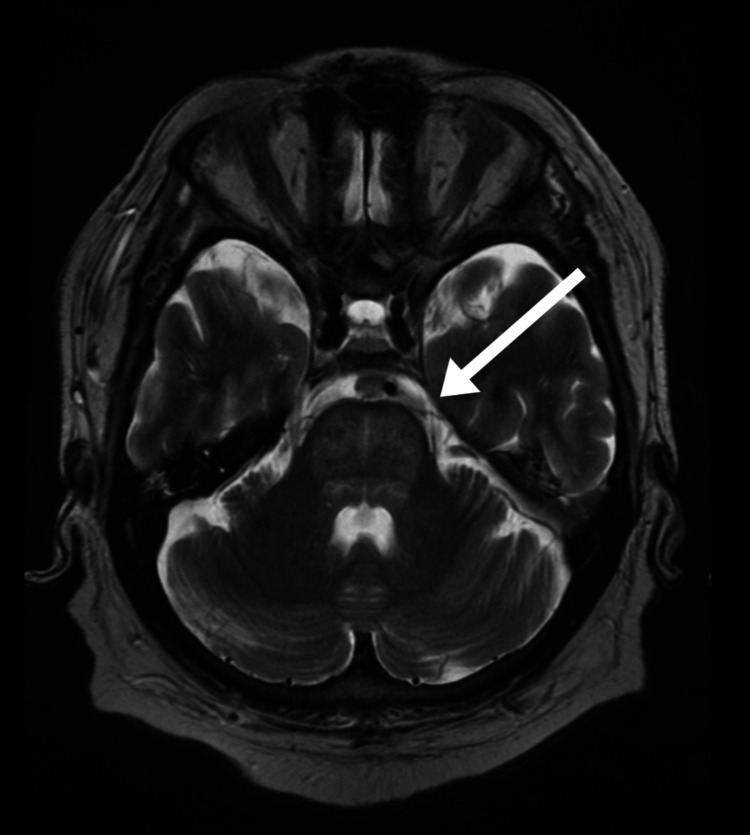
MRI brain showing central pontine hyperintensity on T2/FLAIR FLAIR: fluid-attenuated inversion recovery
